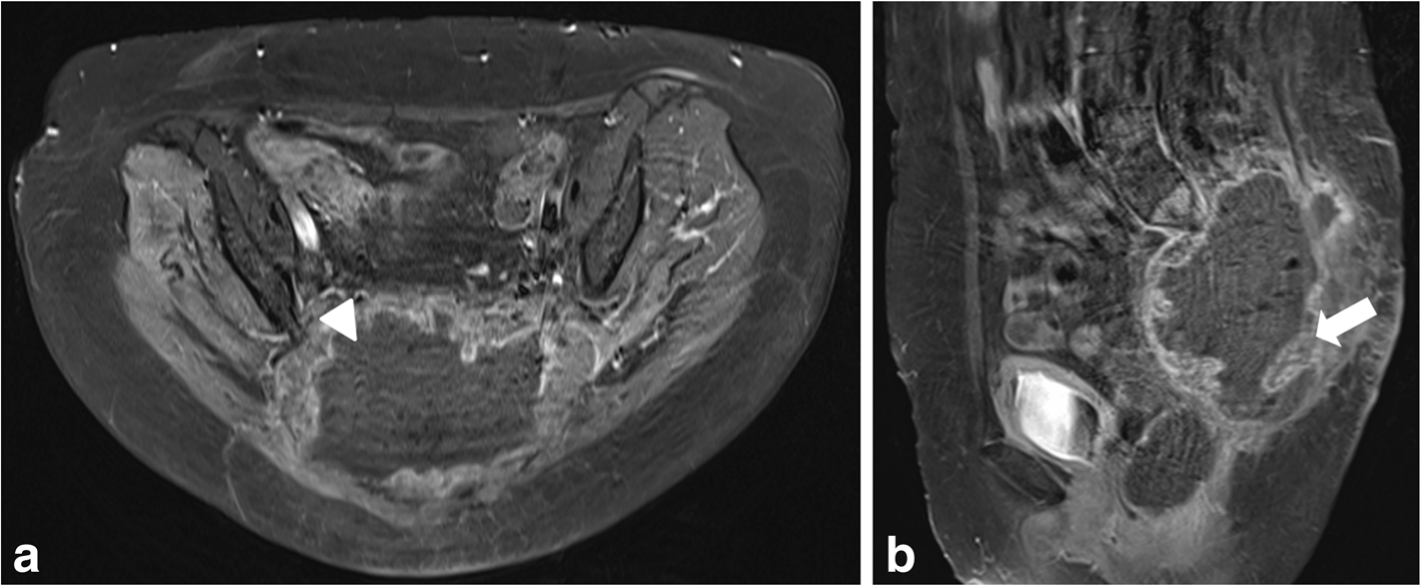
Follow-up postcontrast T1-weighted magnetic resonance images at 8 weeks after second intervention. Only peripheral parts of the tumor mass show viable enhancing tumor tissue (arrow). Central parts of the tumor are necrotic (arrow head). (a: axial plane, b: sagittal plane).
